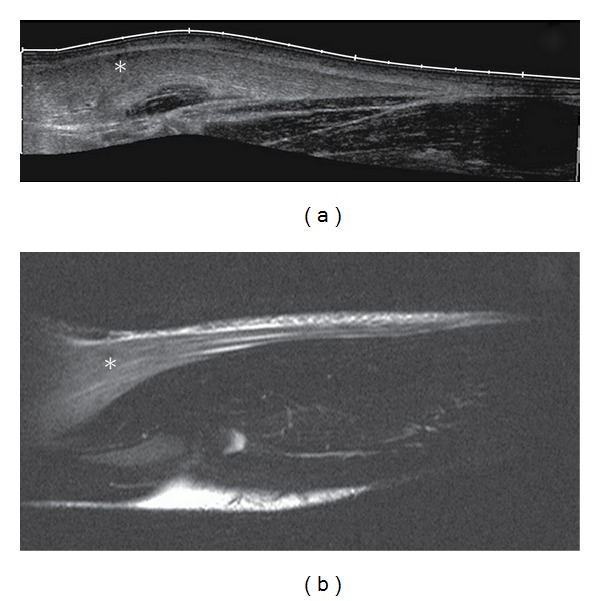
Ultrasound compound image (a) and corresponding sagittal MRI T2w TSE (b) of the right-hand elbow and forearm showing markedly increased echogenicity and corresponding signal increase of the brachioradial muscle (marked as *).
